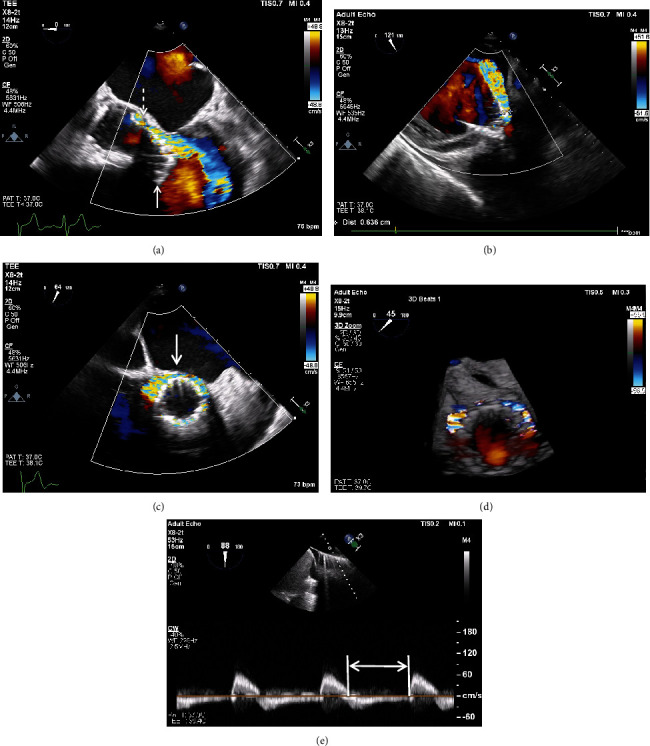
Transesophageal echocardiogram. (a) Aortic bioprosthesis ventricular migration (solid arrow) causing severe PVL (Videos 1–3) by color Doppler (dashed arrow). (b) Transgastric view showing a large vena contracta (0.636 cm). PVL circumferential extent (arrow) involving ~50% of the aortic annulus in the short-axis views in (c) 2D and (d) 3D color Doppler. (e) Thoracic aorta holodiastolic flow reversal consistent with severe PVL (arrow).
